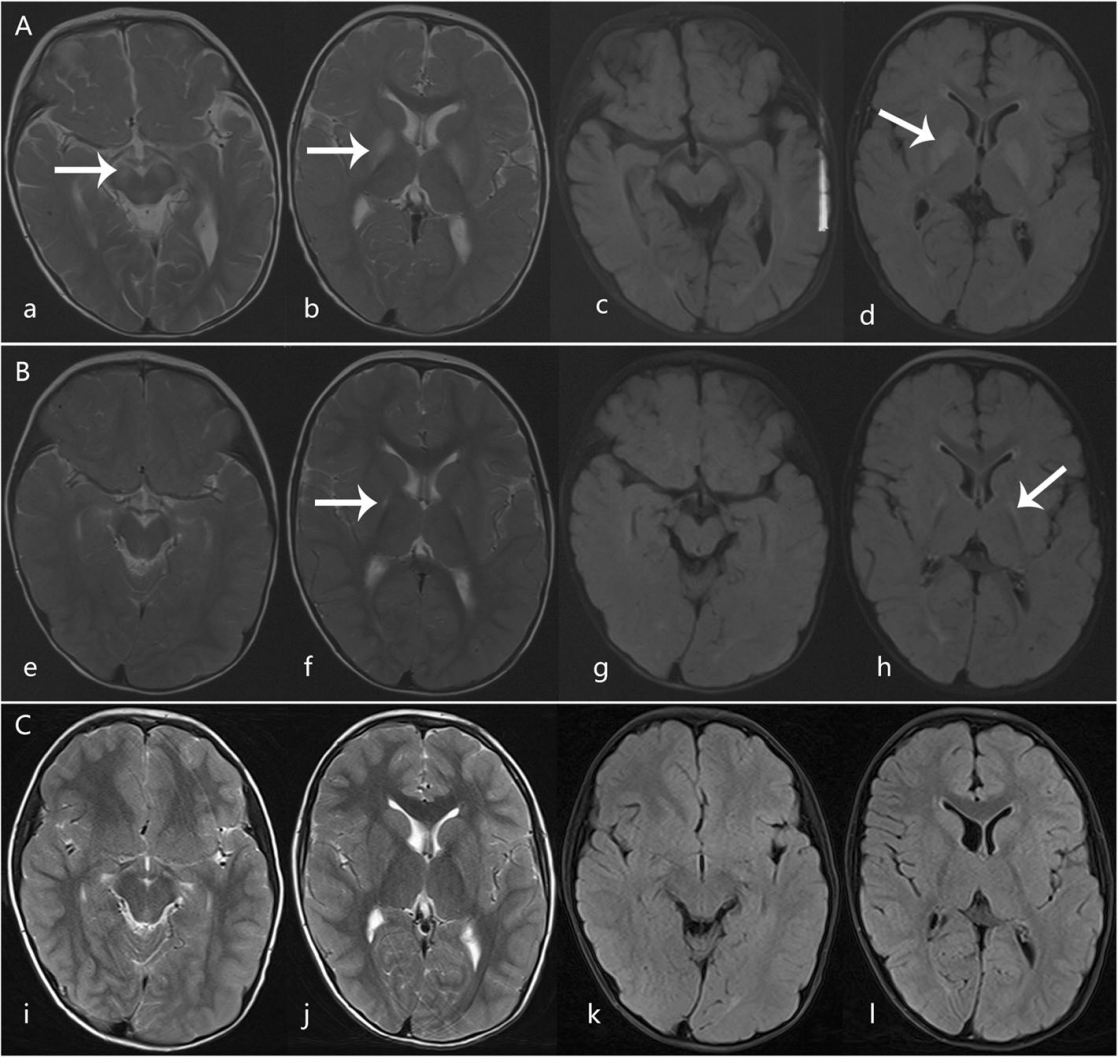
Axial T2-weighted imaging (T2WI) and fluid-attenuated inversion recovery (FLAIR) magnetic resonance imaging (MRI) scans from Case 1. a, b, T2 W1 and c, d, FLAIR performed 3 days after disease onset at age 1, showing high signal in the bilateral globus pallidus and substantia nigra (arrows). e-h, Follow-up images 1 year after onset revealed that hyper-signal lesions significantly shrank (arrows). i-l, Lesions on the basal ganglia had almost disappeared and the lesion on the substantia nigra disappeared 5.5 years after the onset
